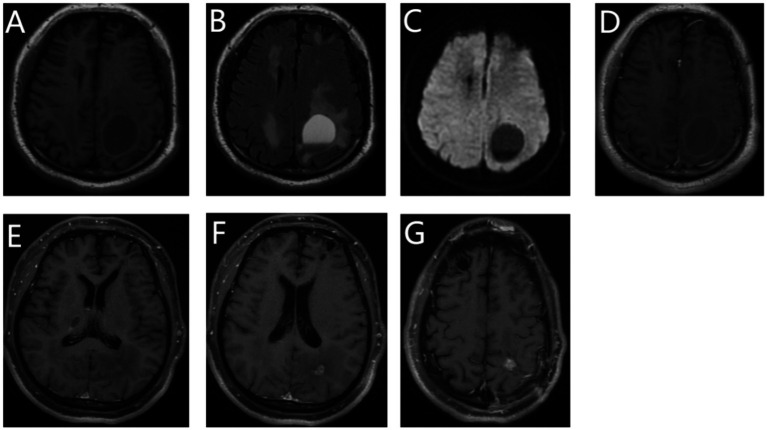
Preoperative cranial MRI of patient 2024 February, and postoperative cranial MRI enhancement 4 months after surgery. (A–D) Preoperative cranial MRI cyst volume increased significantly in February 2024. (E–G) Cranial MRI enhancement in July 2024: cyst disappeared, small nodules were seen in the surgical area.
